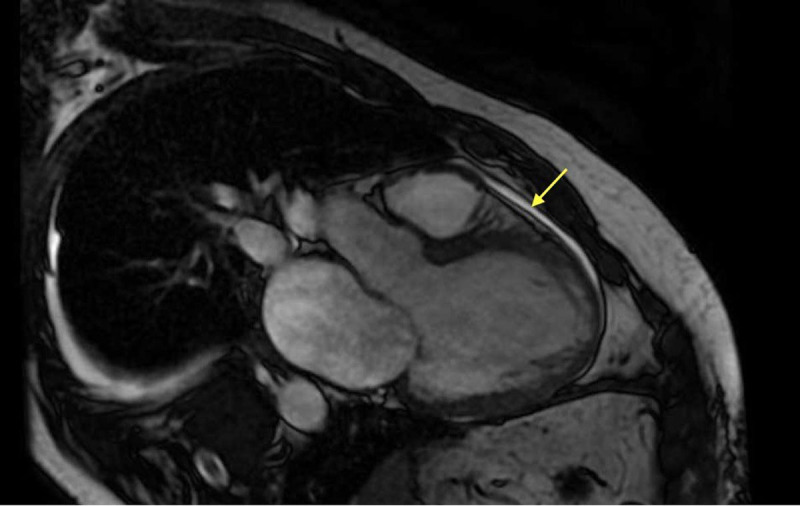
Cardiac magnetic resonance imaging with diffuse late gadolinium enhancement (yellow arrow) consistent with myocardial scarring.
